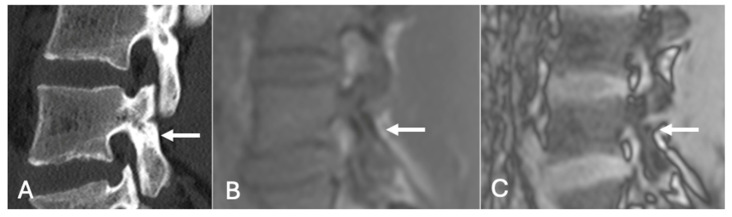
Pars defect assessment sagittal CT image (A) and chemical shift in-phase (B) and out-of-phase (C), showing intact pars of L4 (arrow).
